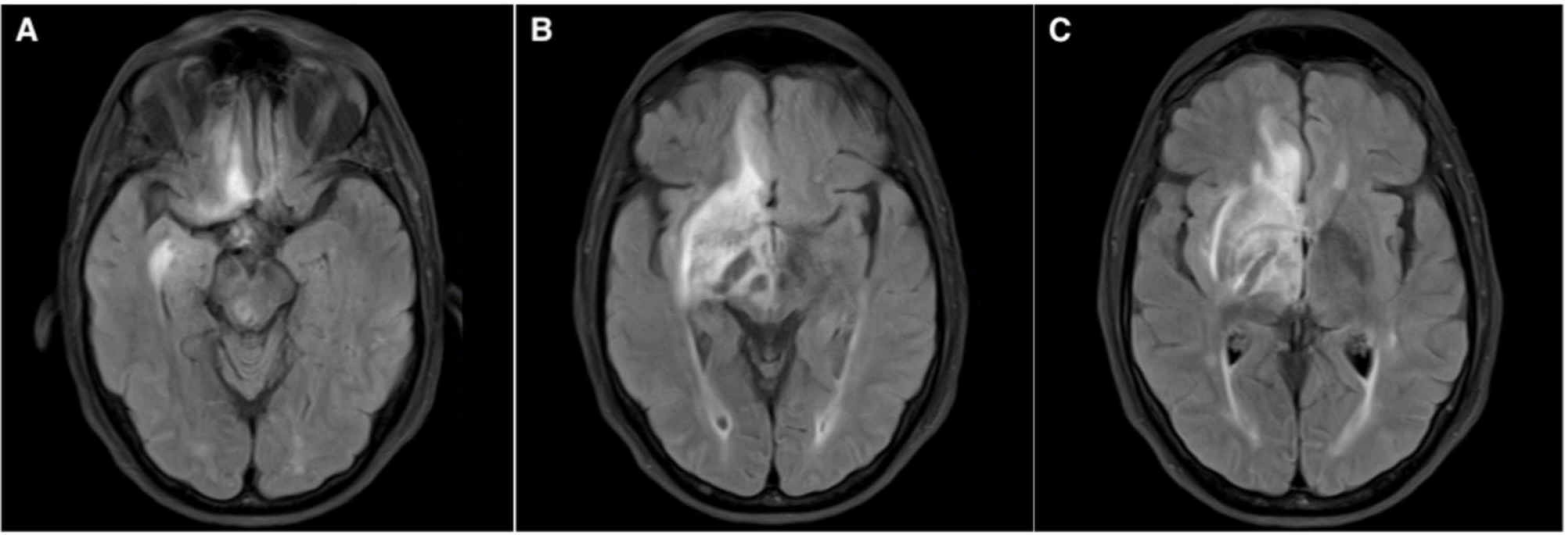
Brain MRI on admission FLAIR MRI sequence on axial plane showing focal hyperintensities in the midbrain (A), basal frontal and temporal lobes (A, B), thalamus and basal ganglia (C) FLAIR, fluid attenuated inversion recovery
